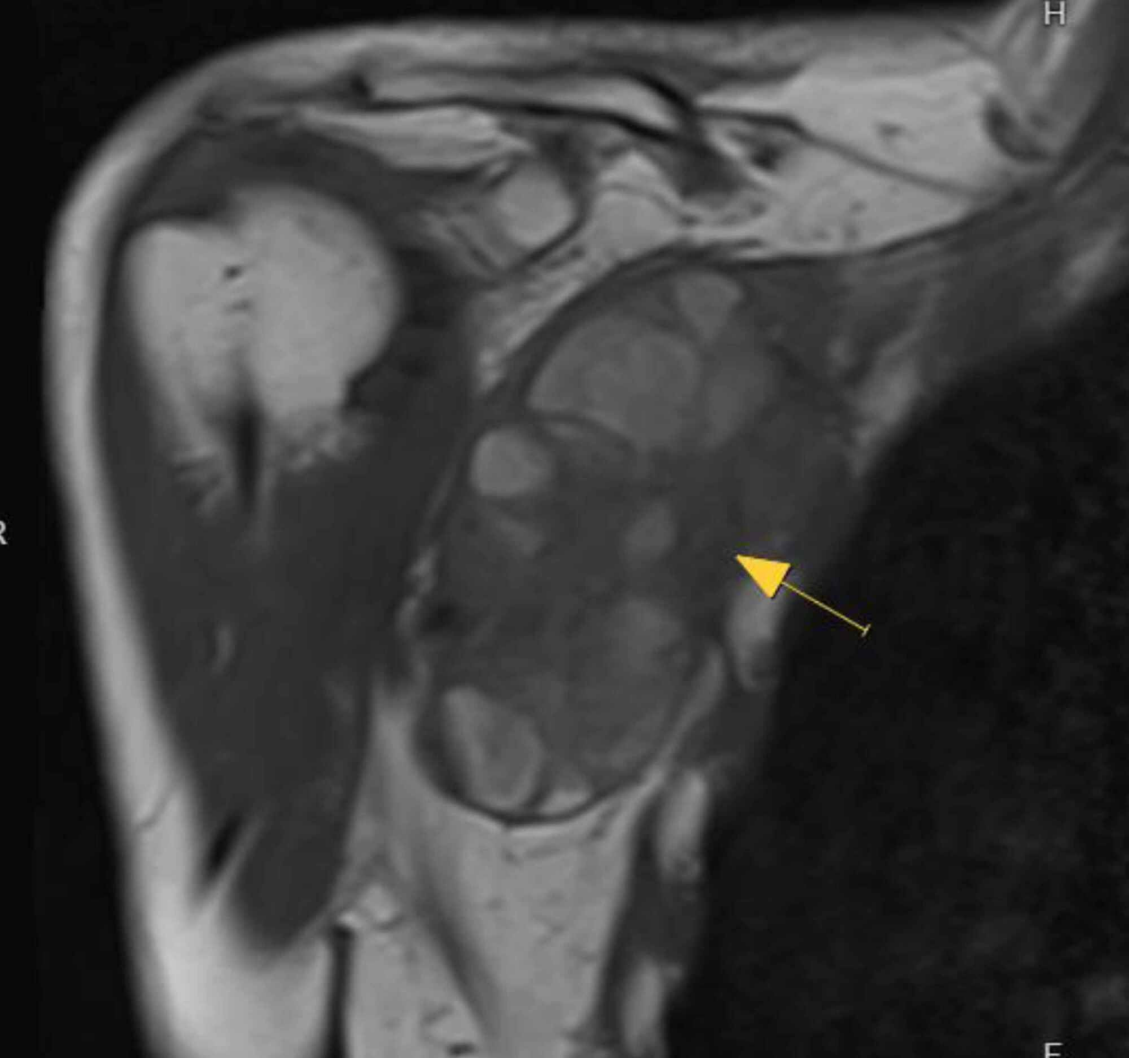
Coronal T1 magnetic resonance imaging of a right axillary malignant peripheral nerve sheath tumor (MPNST).
